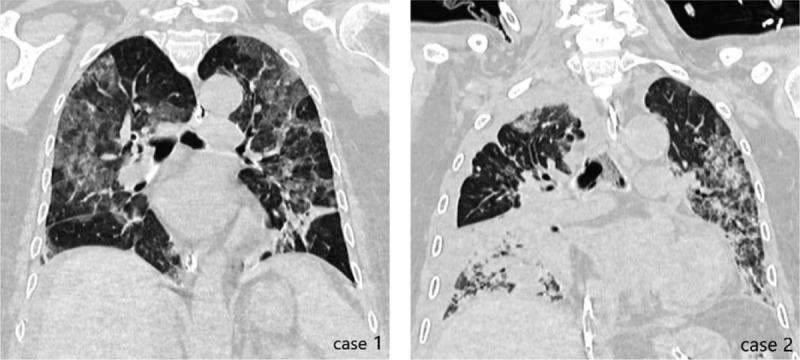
Chest CT scans of case 1 and case 2 when they were intubated for the first time. CT = computed tomography.
